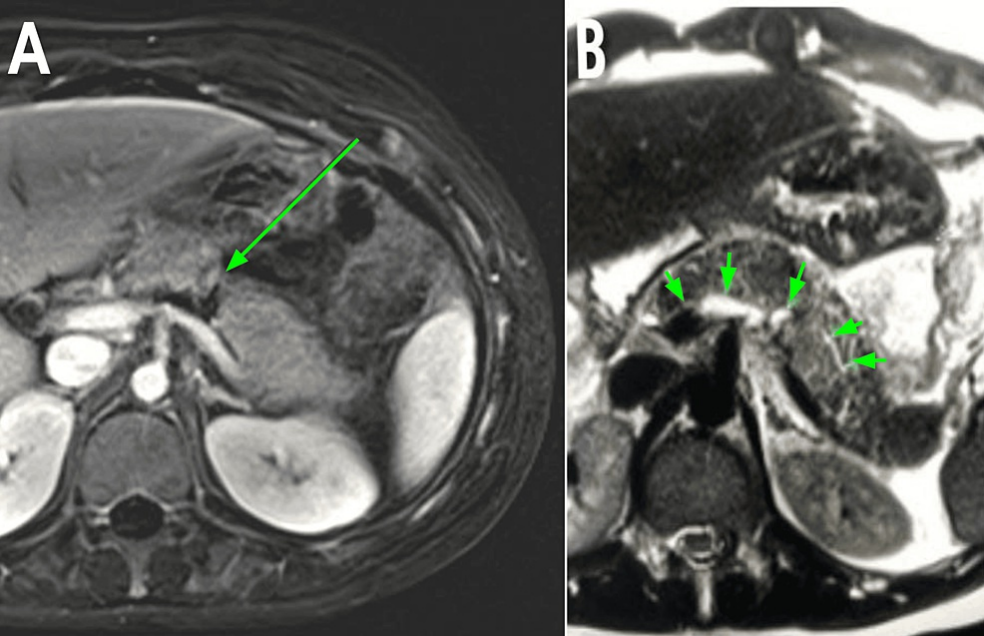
Magnetic resonance image (MRI) with cholangiopancreatography (MRCP). (A) Transection of the entire thickness of the pancreatic parenchyma at the level of the pancreatic body (green arrow). (B) Discontinuity of the PD (green arrows).
